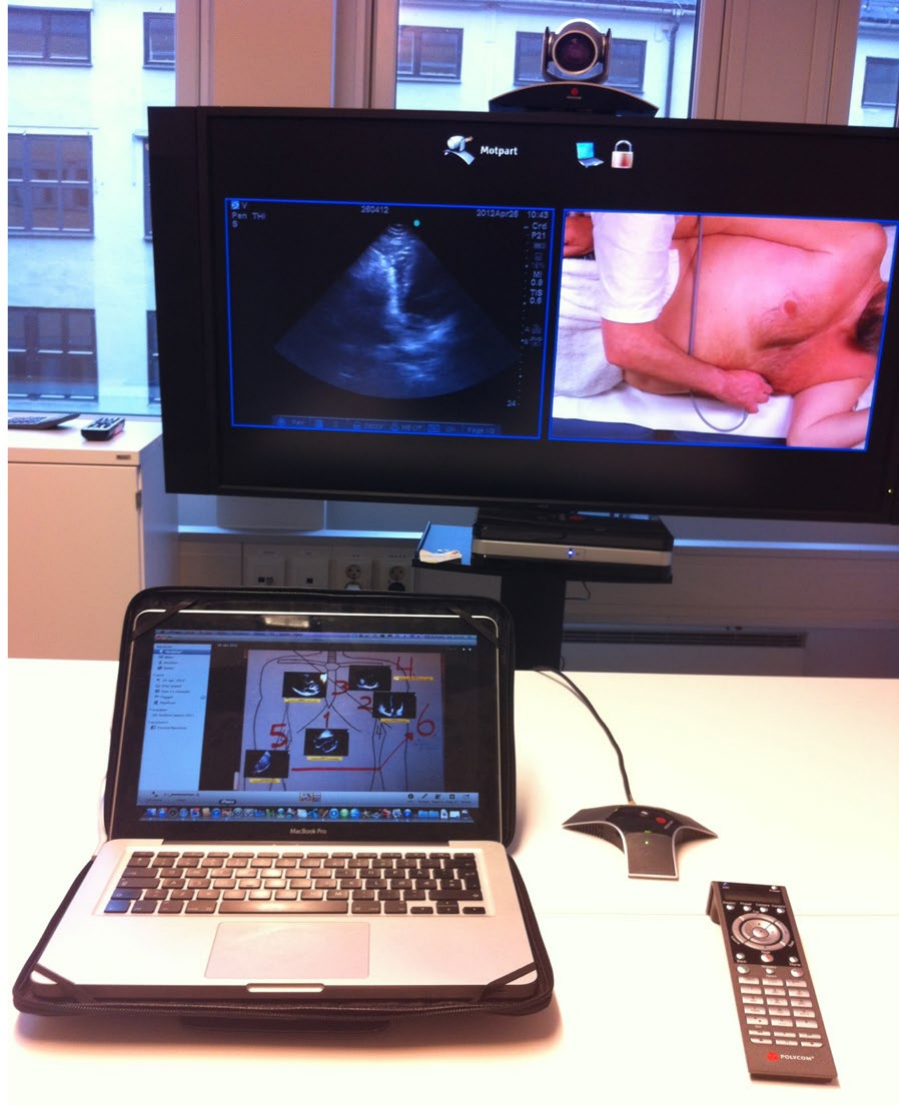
Onshore computer showing the split screen with both the ultrasound image and roof camera video. Photo: Nils Petter Oveland
